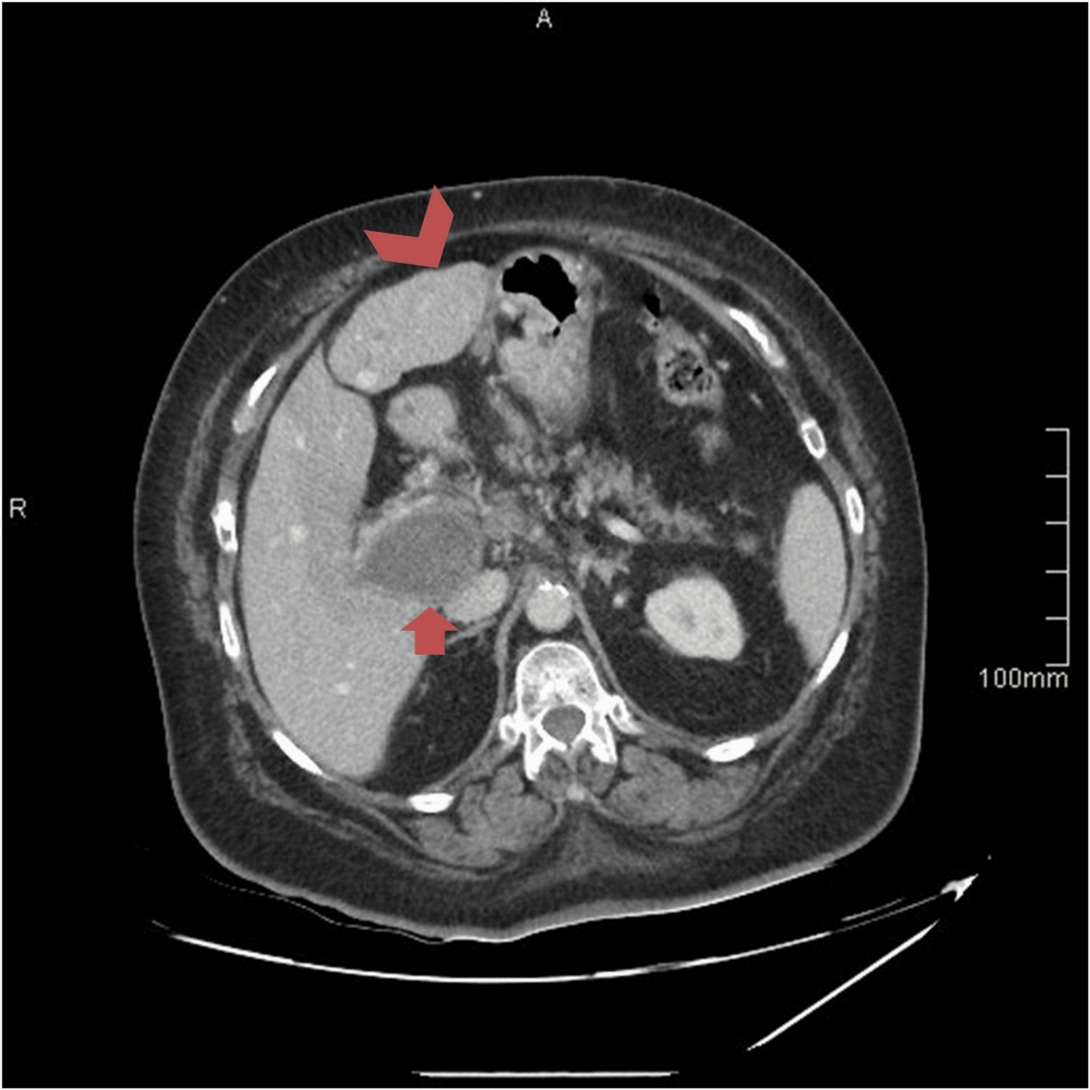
Axial abdominal CT image demonstrating PVT (red arrow) and a mildly lobulated liver contour (red arrowhead) PVT, portal vein thrombosis
